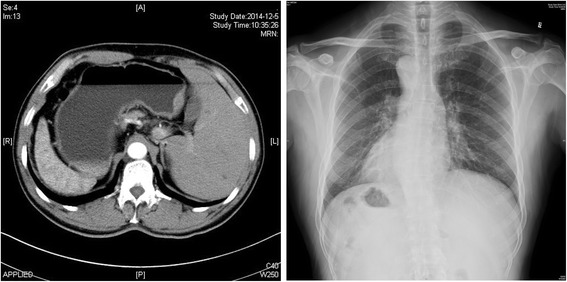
Images of preoperative abdominal CT and a chest X-ray film
